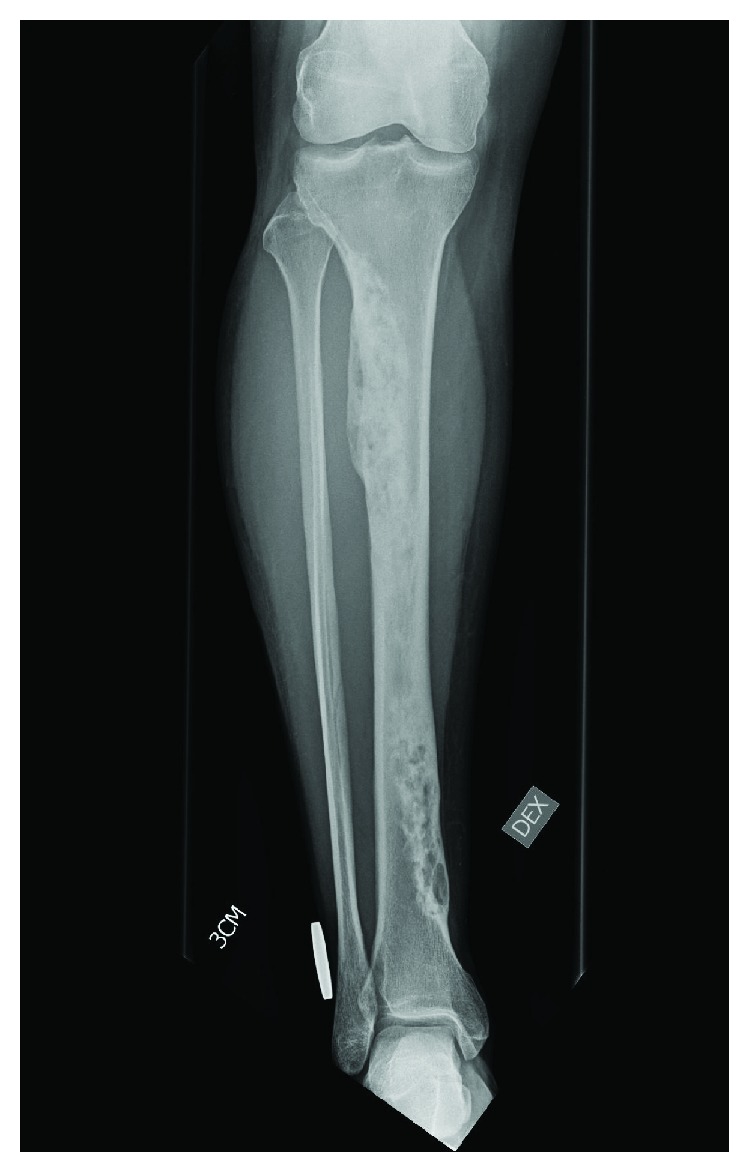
Preoperative X-ray from a right tibia. A plain X-ray from a right tibia with an intraosseal tumor that extended from 4 cm below the knee joint proximally to about 4 cm from the ankle joint distally.
